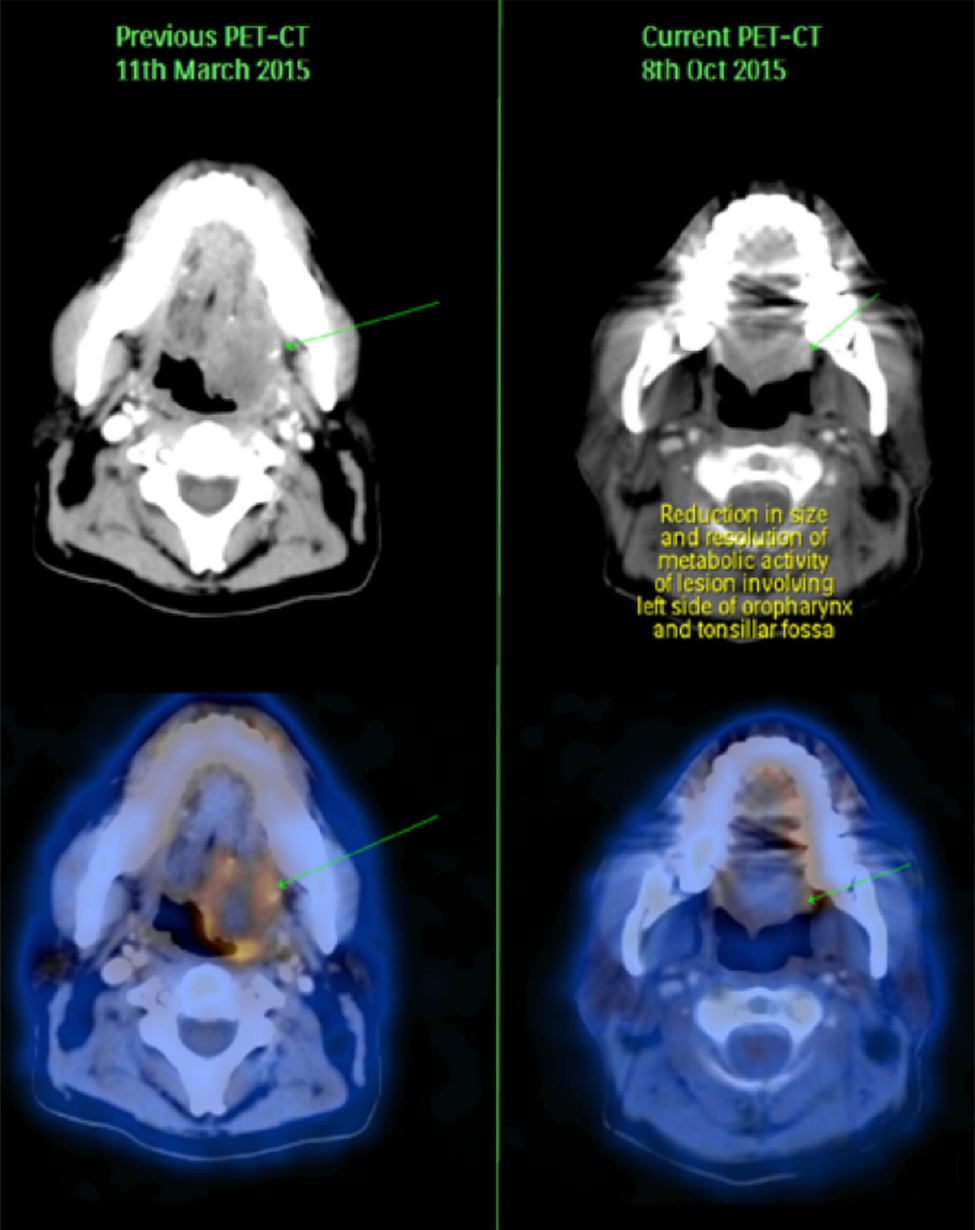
Comparison of PET‐CT scan of the subject conducted on 11/03/2015 (~8 months after last dose of APCEDEN) with PET‐CT scan on 08/10/2015 (~14 months after last dose of APCEDEN). Reduction in tumor size is indicated by green arrow in the image.
